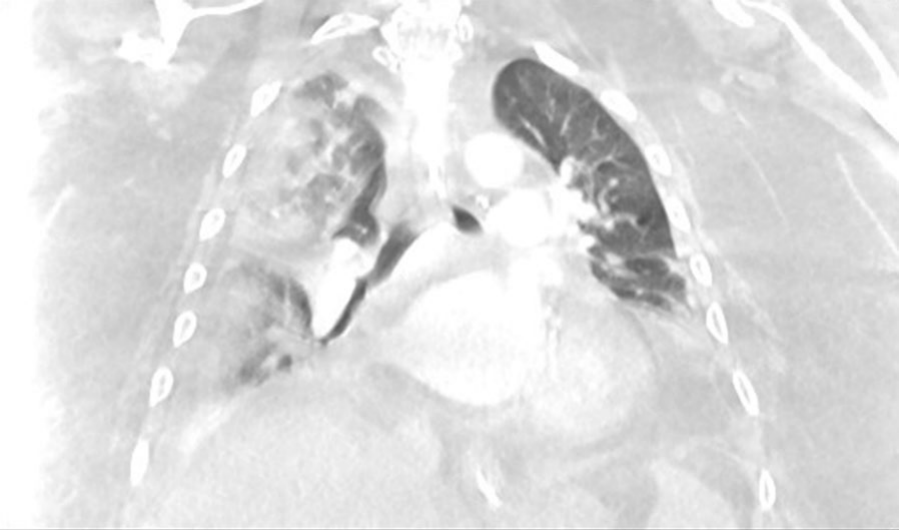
Computed tomography chest coronal view in June 2020
